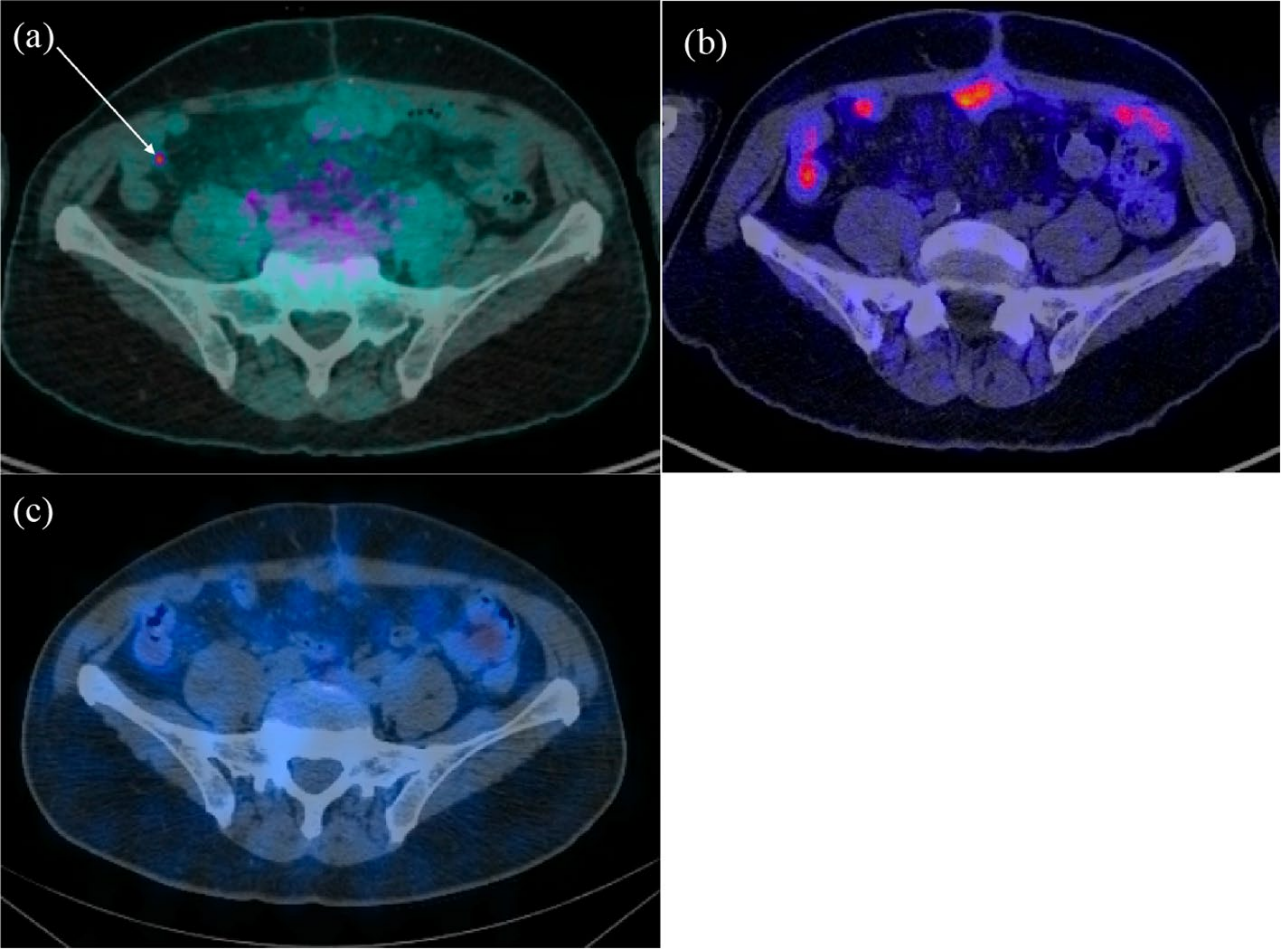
18F-DOPA PET/CT (a), 68Ga-HA-DOTATATE PET/CT (b) and 111In-pentetreotide SPECT/CT (c) axial images of the pelvis. Intensely avid 0.3-cm lymph node within the right lower quadrant mesentery adjacent to the neoterminal ileum demonstrated intense 18F-DOPA activity (a), but no 68Ga-HA-DOTATATE (b) or 111In-pentetreotide (c) avidity
